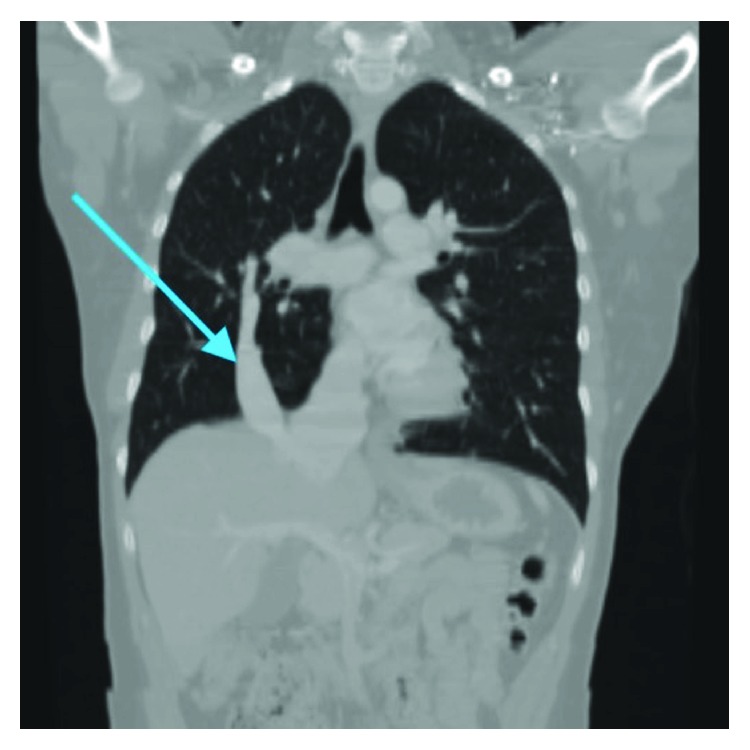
Coronal CT with IV contrast. Arrow pointing to scimitar vein in the right lower lobe, which drains into the IVC.
